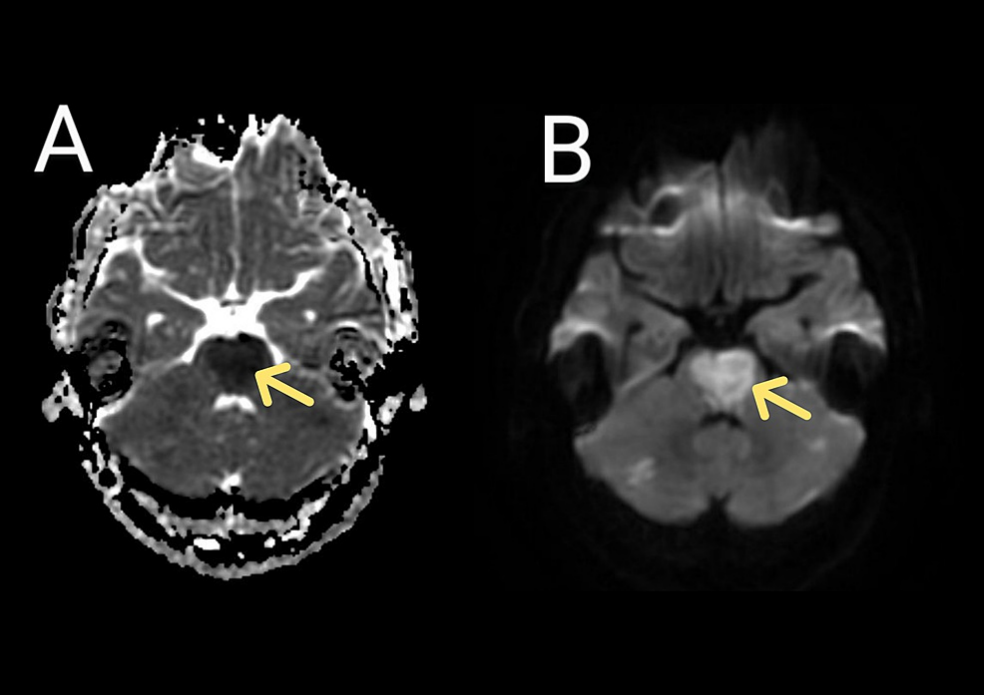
Two scans of magnetic resonance imaging of the brain, yellow arrows point towards the infarct in the brainstem A: Apparent diffusion coefficient (ADC) showing extensive brainstem infarct. B: Diffusion-weighted imaging (DWI) sequence showing brainstem.
